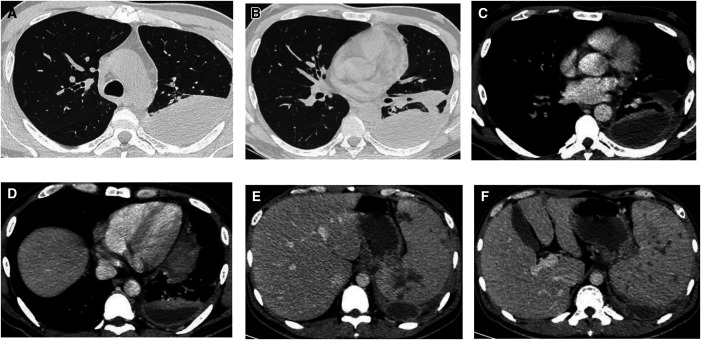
Initial computed tomography of the thoracic and abdominal regions. (A–D) Infectious lesions in the left lung, primarily in the lower lobe, with partial consolidation and left-sided encapsulated pleural effusion. (E, F) Weakly enhanced shadows in the spleen and a low-density shadow posterior to the spleen.
